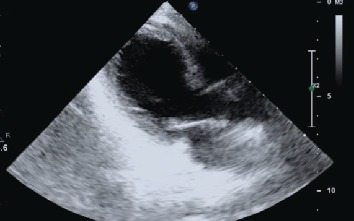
Echocardiogram long axis view revealed apical ballooning of the LV.
